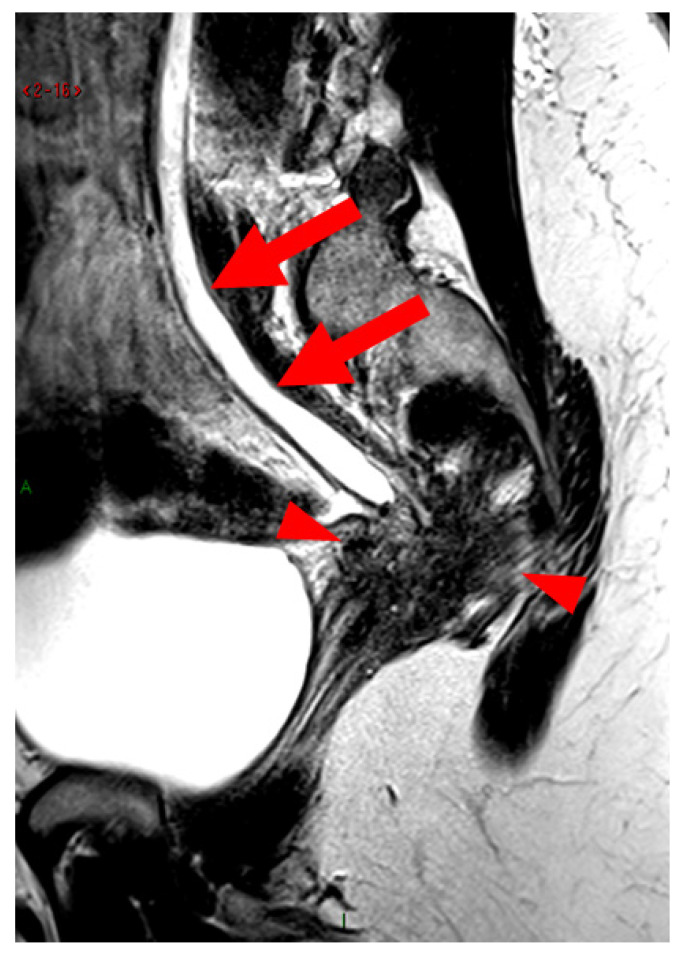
Pelvic MRI scan of a patient with HTD type 6 USLs. Sagittal T2WI demonstrates nodulospicular infiltration of the sacro-recto-genital septum, with the endometriotic lesion delineating the structure of the inferior hypogastric plexus (*arrowheads*). Note that the lesion also involves the distal ureter, causing upstream ureteral dilation (*arrows*).
